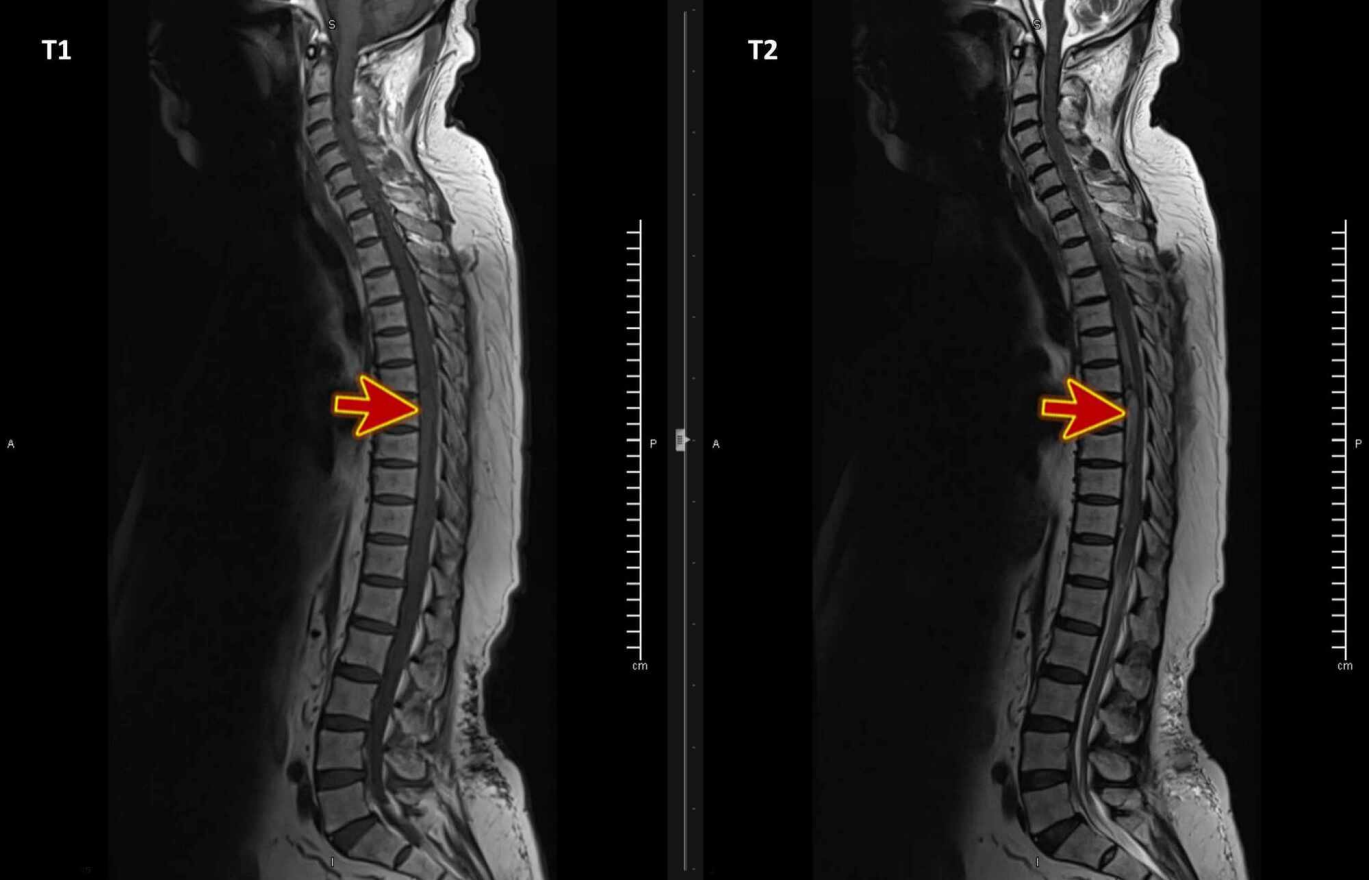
MRI T1WI and T2WI of the whole spines show extensive extradural hematoma (red arrows) extending from the foramen magnum to the fifth lumbar vertebral level (L5) MRI: magnetic resonance imaging, T1WI: T1-weighted image, T2WI: T2-weighted image.
